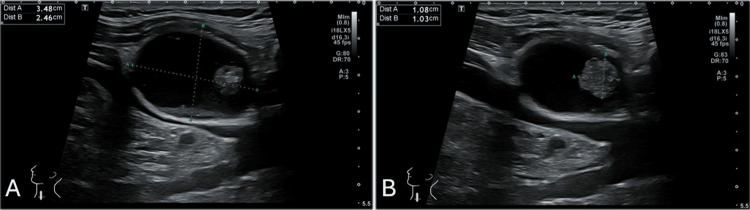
Preoperative ultrasound of the left level IV cervical region demonstrating a complex cystic lymph node. (A) Predominantly cystic component measuring approximately 3.5×3×2.5 cm. (B) Internal solid, vascularized component measuring approximately 1.1×1×1 cm, targeted on repeat fine-needle aspiration and diagnostic of papillary thyroid carcinoma
